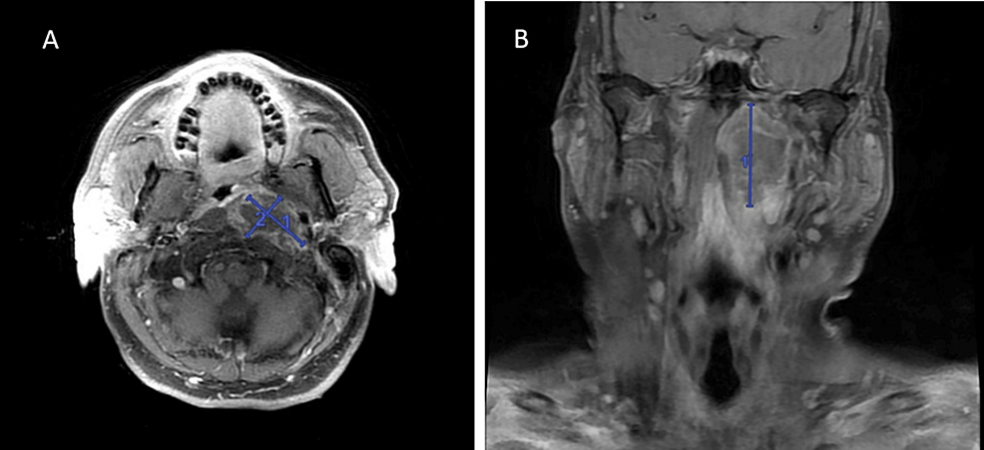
MRI head and neck trans-spatial, rim-enhancing fluid collection centered within the left parapharyngeal space, measuring 51 x 38 x 27 mm
